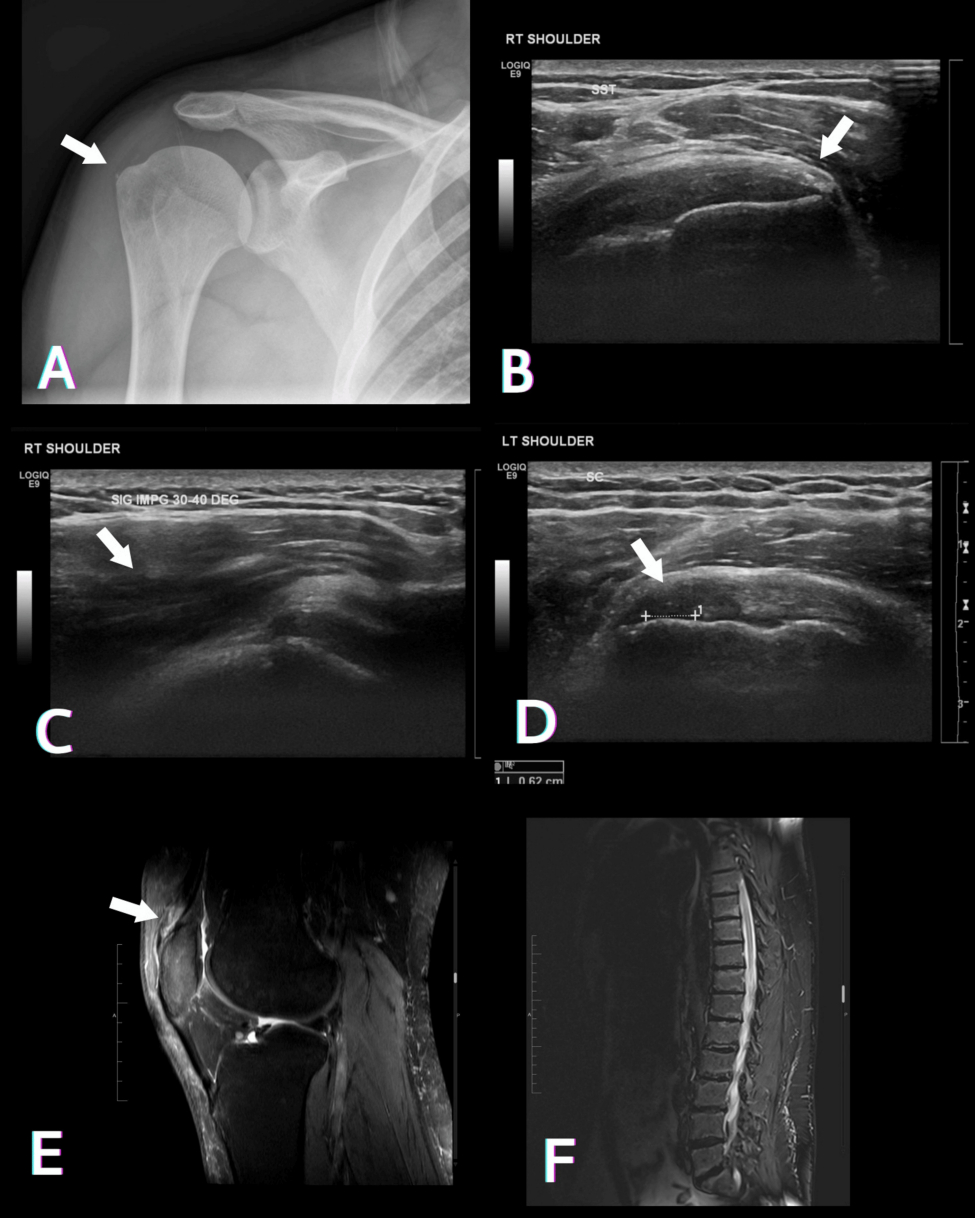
Radiological findings of several presentations of multiple joint pain. (A) Plain X-ray of the right shoulder showing calcifications at the insertion of the supraspinatus tendon. (B) Right shoulder ultrasonography showing calcifications at the insertion of the supraspinatus tendon. (C) Right shoulder ultrasonography showing significant limitation of abduction and positive subacromial impingement, with limited abduction at 30-40 degrees. (D) Left shoulder ultrasonography showing a stable partial thickness tear of the subscapularis tendon. (E) Left knee MRI sagittal PD FS showing quadriceps tendinopathy and partial tear. (F) Sagittal view of thoracolumbar spine MRI STIR showing multilevel degenerative changes of the lower thoracic and lumbar spine, including end plate changes and intervertebral disc degenerative changes.
